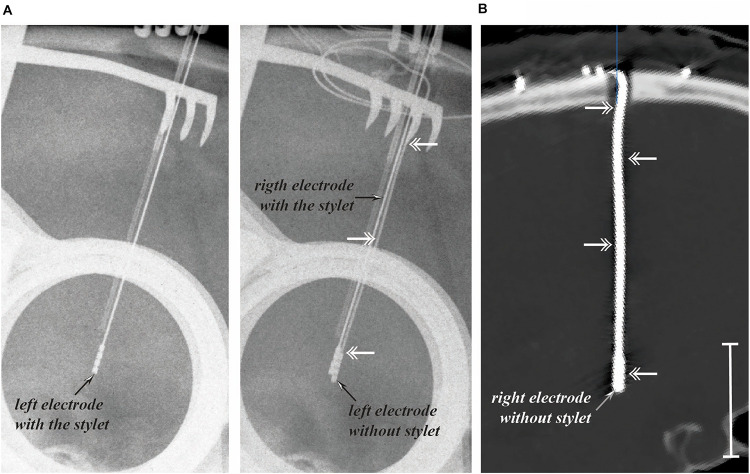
Electrode deformation following stylet retraction. Intraoperative X-rays (A; lateral projection; anterior is on the right) showing the electrode deformation (double arrows) after removal of stylet (left and intermediate rows) and immediately after removal of the stereotactic frame on postoperative CT scan (B; pseudo sagittal reconstructed slice along the right electrode; anterior is on the right; white vertical bar = 25 mm).
